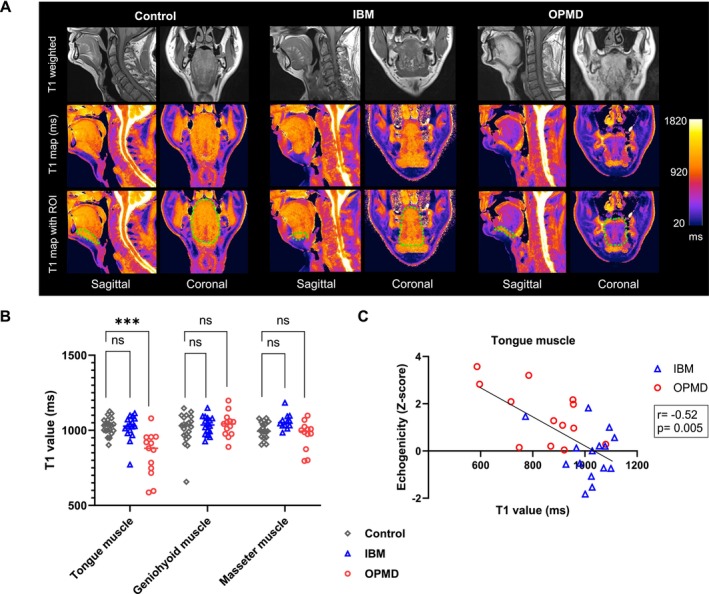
T1 mapping of swallowing muscles in patients with IBM and OPMD versus control subject. (A) Selected T1 weighted MRI sequences (upper row) and T1 maps (middle and bottom row) obtained for head and neck region of a control subject, an IBM patient and an OPMD patient, all male and between 61 and 71 years old. The bottom row outlines the manually placed regions of interest (dotted green lines) of the tongue muscles in the coronal sequences and the geniohyoid muscles in the sagittal sequences for analysis of mean T1 values. (B) T1 mapping reveal significantly lower T1 values of tongue muscle in OPMD patients, indicating a higher fat content. T1 values of geniohyoid muscle and masseter muscle show no significant differences between patients and controls. Variables were tested using *t*‐test. *p* values were adjusted for multiplicity using the Holm–Šidák method, significance is defined as *p* ≤ 0.05. (C) The reduced T1 values of the tongue in quantitative MRI correlate significantly with increased echogenicity of the tongue on ultrasound in OPMD patients (Spearman's *r* = −0.52; *p* = 0.005), both methods thereby indicate fibrosis and fatty infiltration of the tongue muscle.
